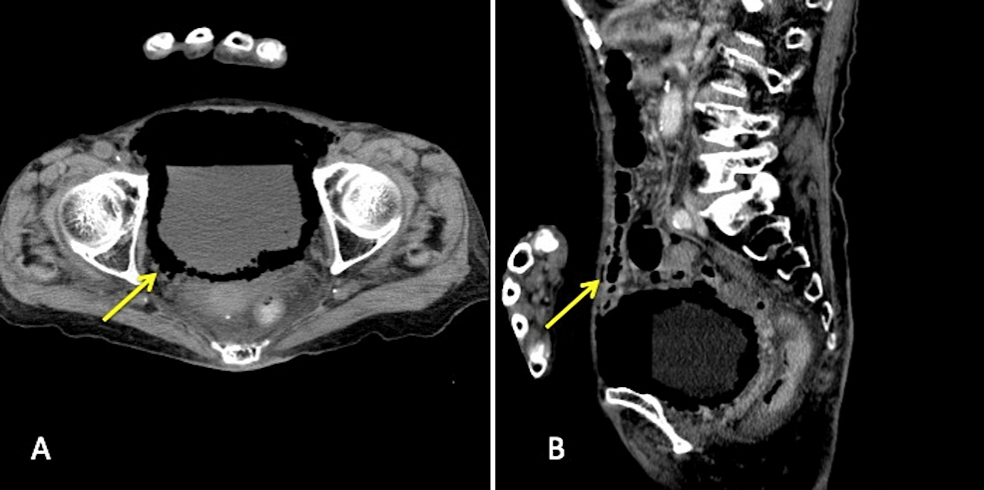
Contrast-enhanced computed tomography performed on day one A: Axial image. Multiple gas pockets are present within the bladder wall, consistent with emphysematous cystitis (arrow). No ascites was present. B: Sagittal image. Free air is present beneath the anterior abdominal wall (arrow).
